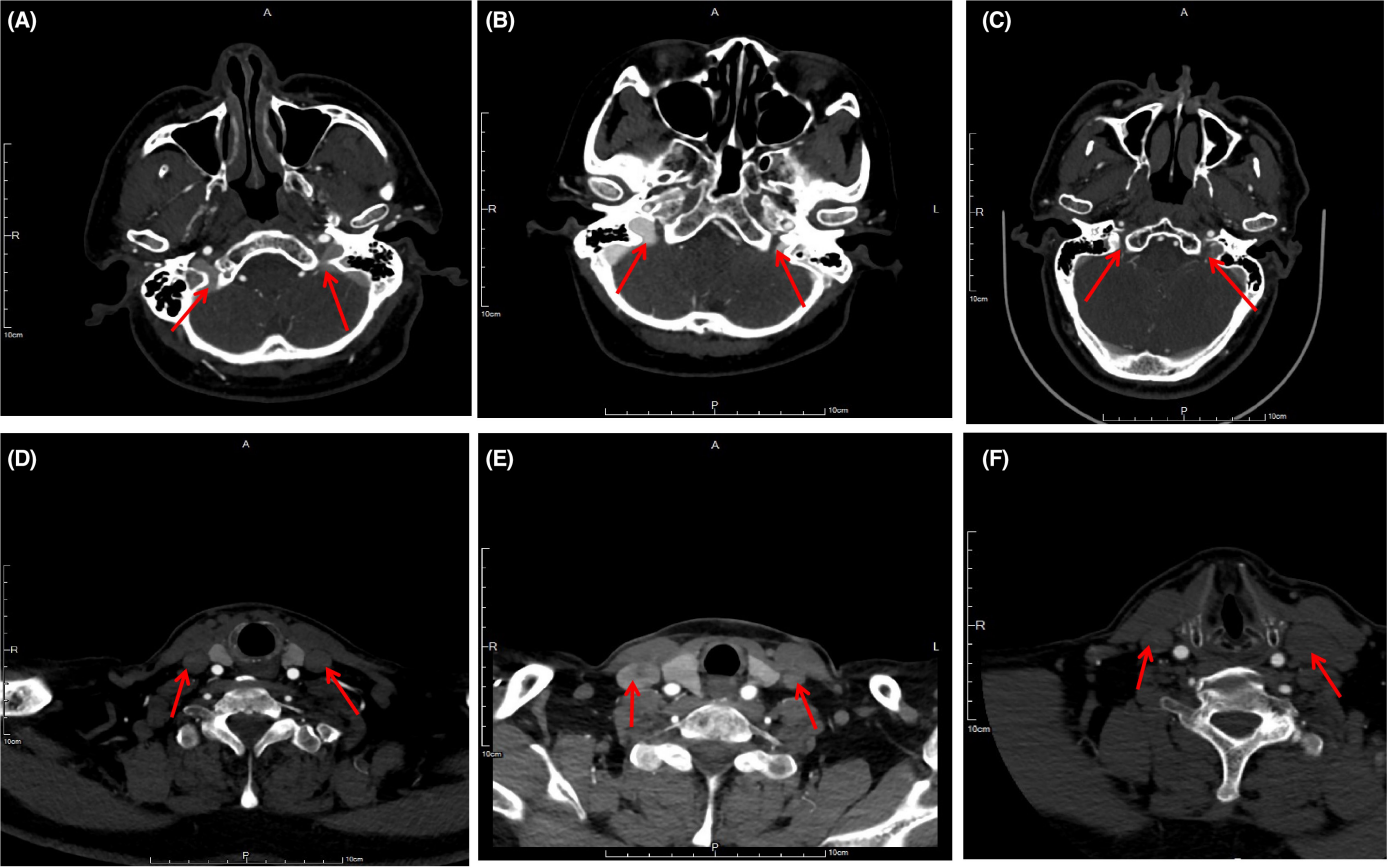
Modalities of internal jugular foramens on CT (red arrow): A: left larger‐aperture, B: right larger‐aperture, C: bilateral symmetry
